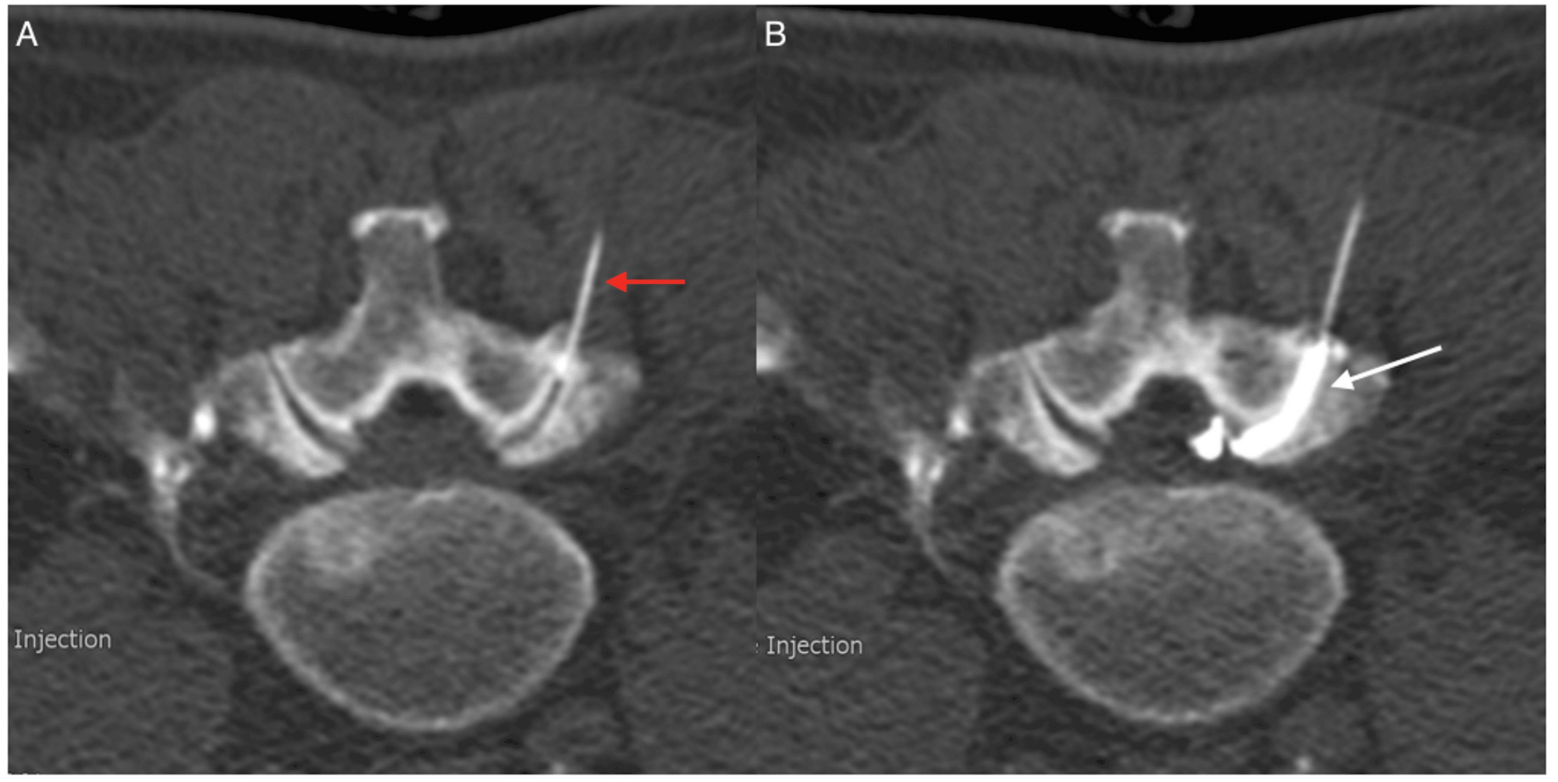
Axial CT images (A) A spinal needle (red arrow) is inserted into the right L4/L5 facet joint and contrast is injected through the right L4/L5 facet joint. (B) The contrast fills the cyst and causes its rupture (white arrow).
